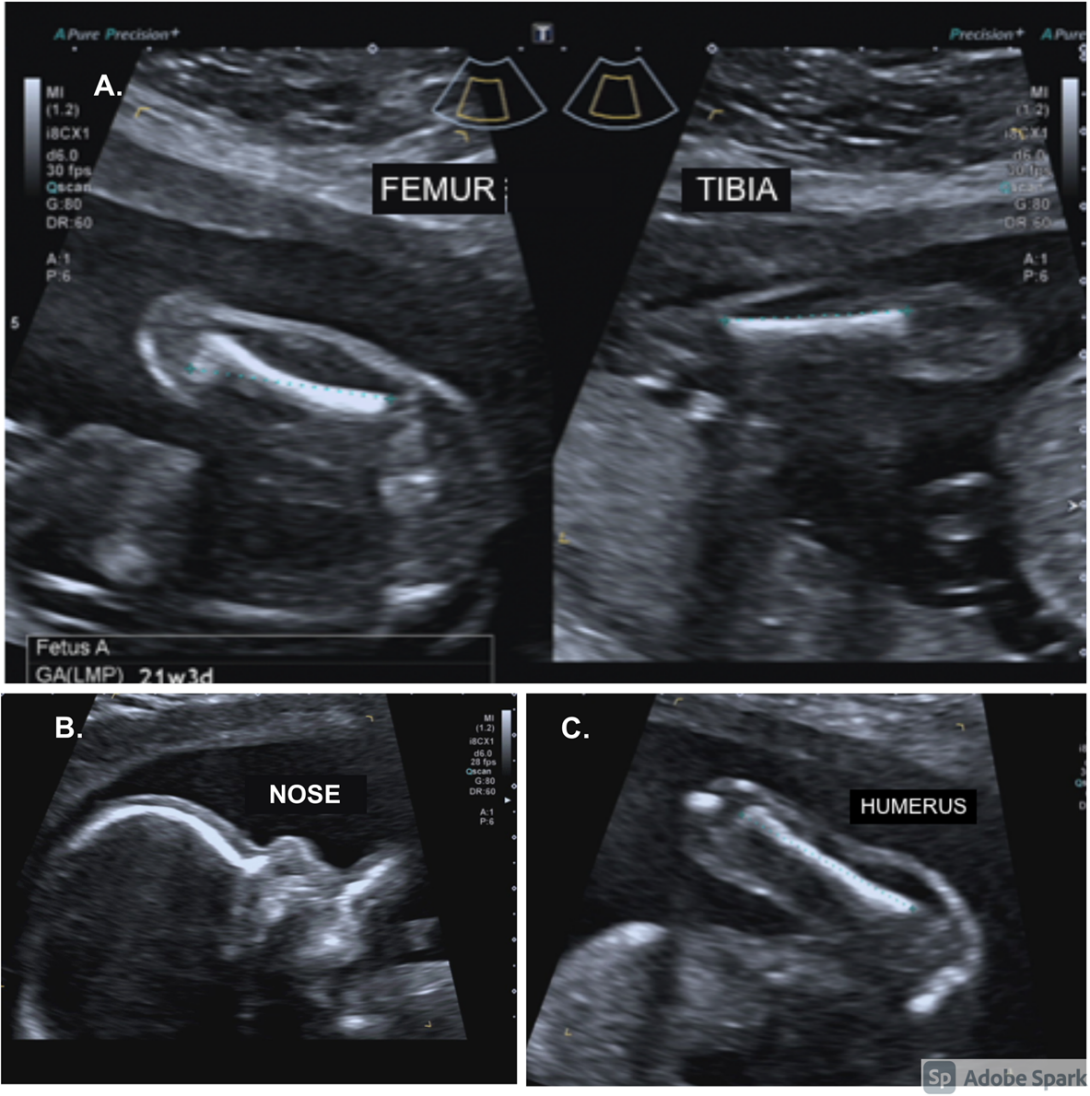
Ultrasounds skeletal findings. A. Femur and tibia< 5° centile B. Prominence of the forehead with mild nasal hypoplasia. C. Homerus 5° centile
